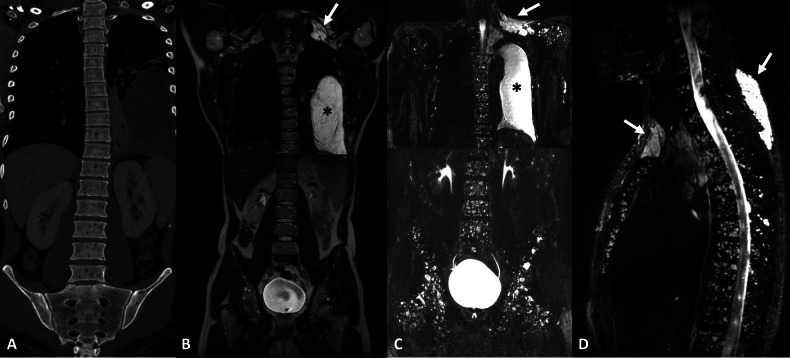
Initial work-up of a posterior cervical mass in a 26-year-old male revealing lymphangiomatosis (GLA) with multiple intraosseous cystic lymphangiomas of the axial skeleton. These multiple bone lesions did not cause any pain. A Post-contrast CT, coronal view. Multiple round lytic bone lesions, with regular and well-defined borders, without cortical destruction, located on the axial skeleton. B T2w TSE, coronal view. The multiple intramedullary lesions seen on CT exhibit homogeneous high signal intensity. There is no associated bone marrow edema. C, D 3D FRFSE T2w, MIP reconstruction (20 mm), coronal (C) and sagittal (D) views. NEMRL reveals associated lymphatic anomalies, including soft-tissue cystic lymphangiomas (arrows) of the left supra-clavicular space, superior mediastinum, posterior cervical area, and the left chylothorax (*). Note how the countless intraosseous cystic lymphangiomas are also well identified
